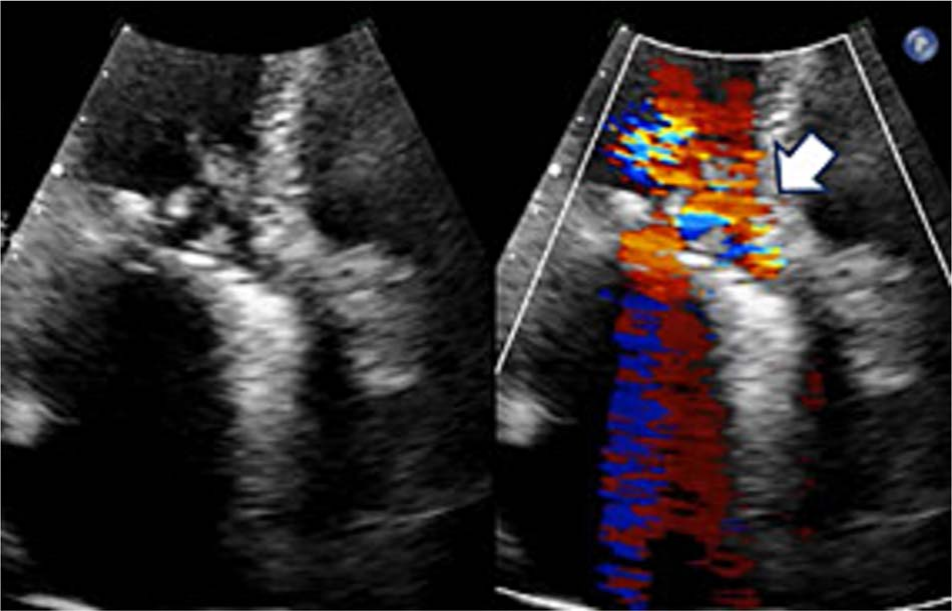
Preoperative transthoracic echocardiography: significant paraventricular leakage was shown with the white arrow.
